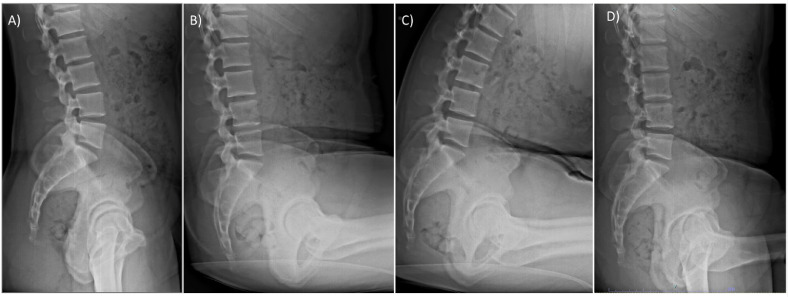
(A) Standing (B) Sitting, (C) Flexed forward, and (D) Single-leg step up radiographs.
